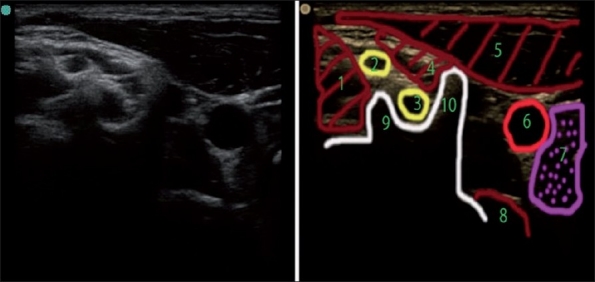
Lateral axial ultrasound neck image at the upper pole of thyroid (C6 vertebral level). (1) Middle scalene, (2) C5 nerve root, (3) C6 nerve root, (4) anterior scalene, (5) sternocleidomastoid, (6) carotid artery, (7) thyroid, (8) longus colli, (9) posterior tubercle of C6, (10) anterior tubercle of C6 (carotid tubercle)
